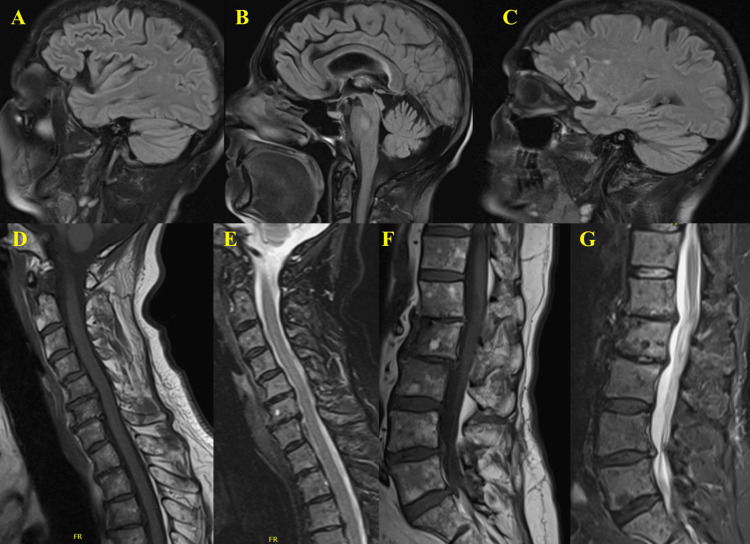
MRI of the brain, cervical spine, and lumbar spine. Brain MRI (images A-C) demonstrates no acute infarct, intracranial hemorrhage, or mass effect, with white matter changes consistent with chronic small vessel ischemia and no evidence of demyelination or abnormal enhancement, with image A representing the right side, image B the midline, and image C the left side; cervical spine MRI demonstrates moderate multilevel spondylosis with mild canal stenosis and no cord signal abnormality or abnormal cord enhancement, with image D representing a T1-weighted sequence and image E representing a T2-weighted sequence; and lumbar spine MRI demonstrates no abnormal enhancement or critical canal stenosis, with image F representing a T1-weighted sequence and image G representing a T2-weighted sequence.
